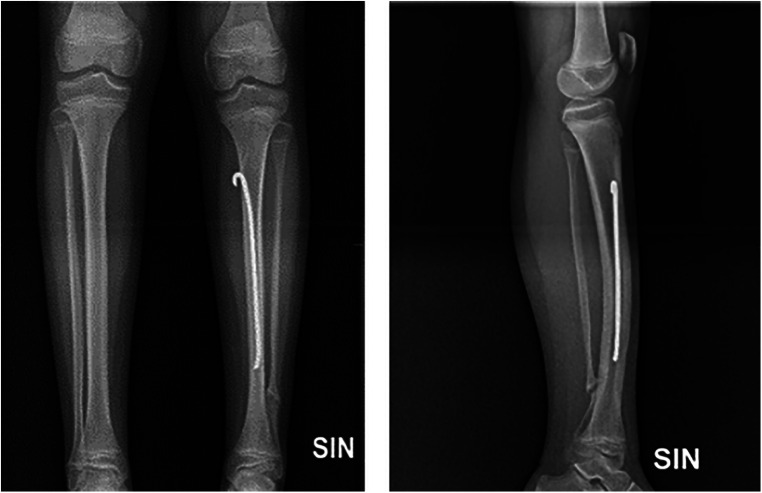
X-ray after eight-year follow-up. The rush rod is far away from the distal tibial cartilage growth plate. The tibial osteotomy site is perfectly healed. No growth plate disturbances are present.
